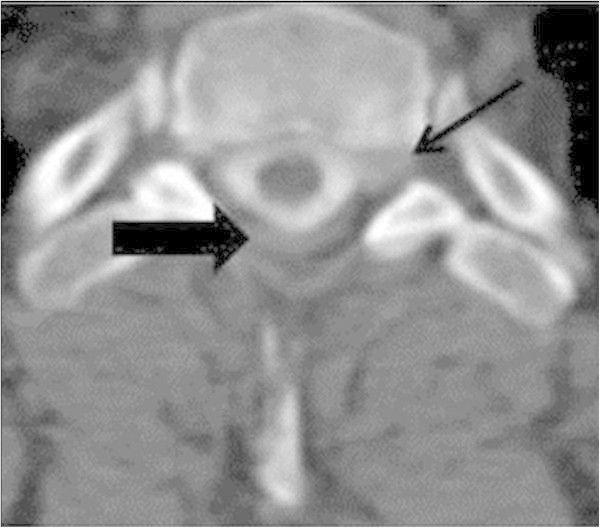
Case I: Axial Myelo- CT presenting the point of leakage on the left side, D1 nerve (narrow 1) and the indirect leakage around the spinal cord (narrow 2).
